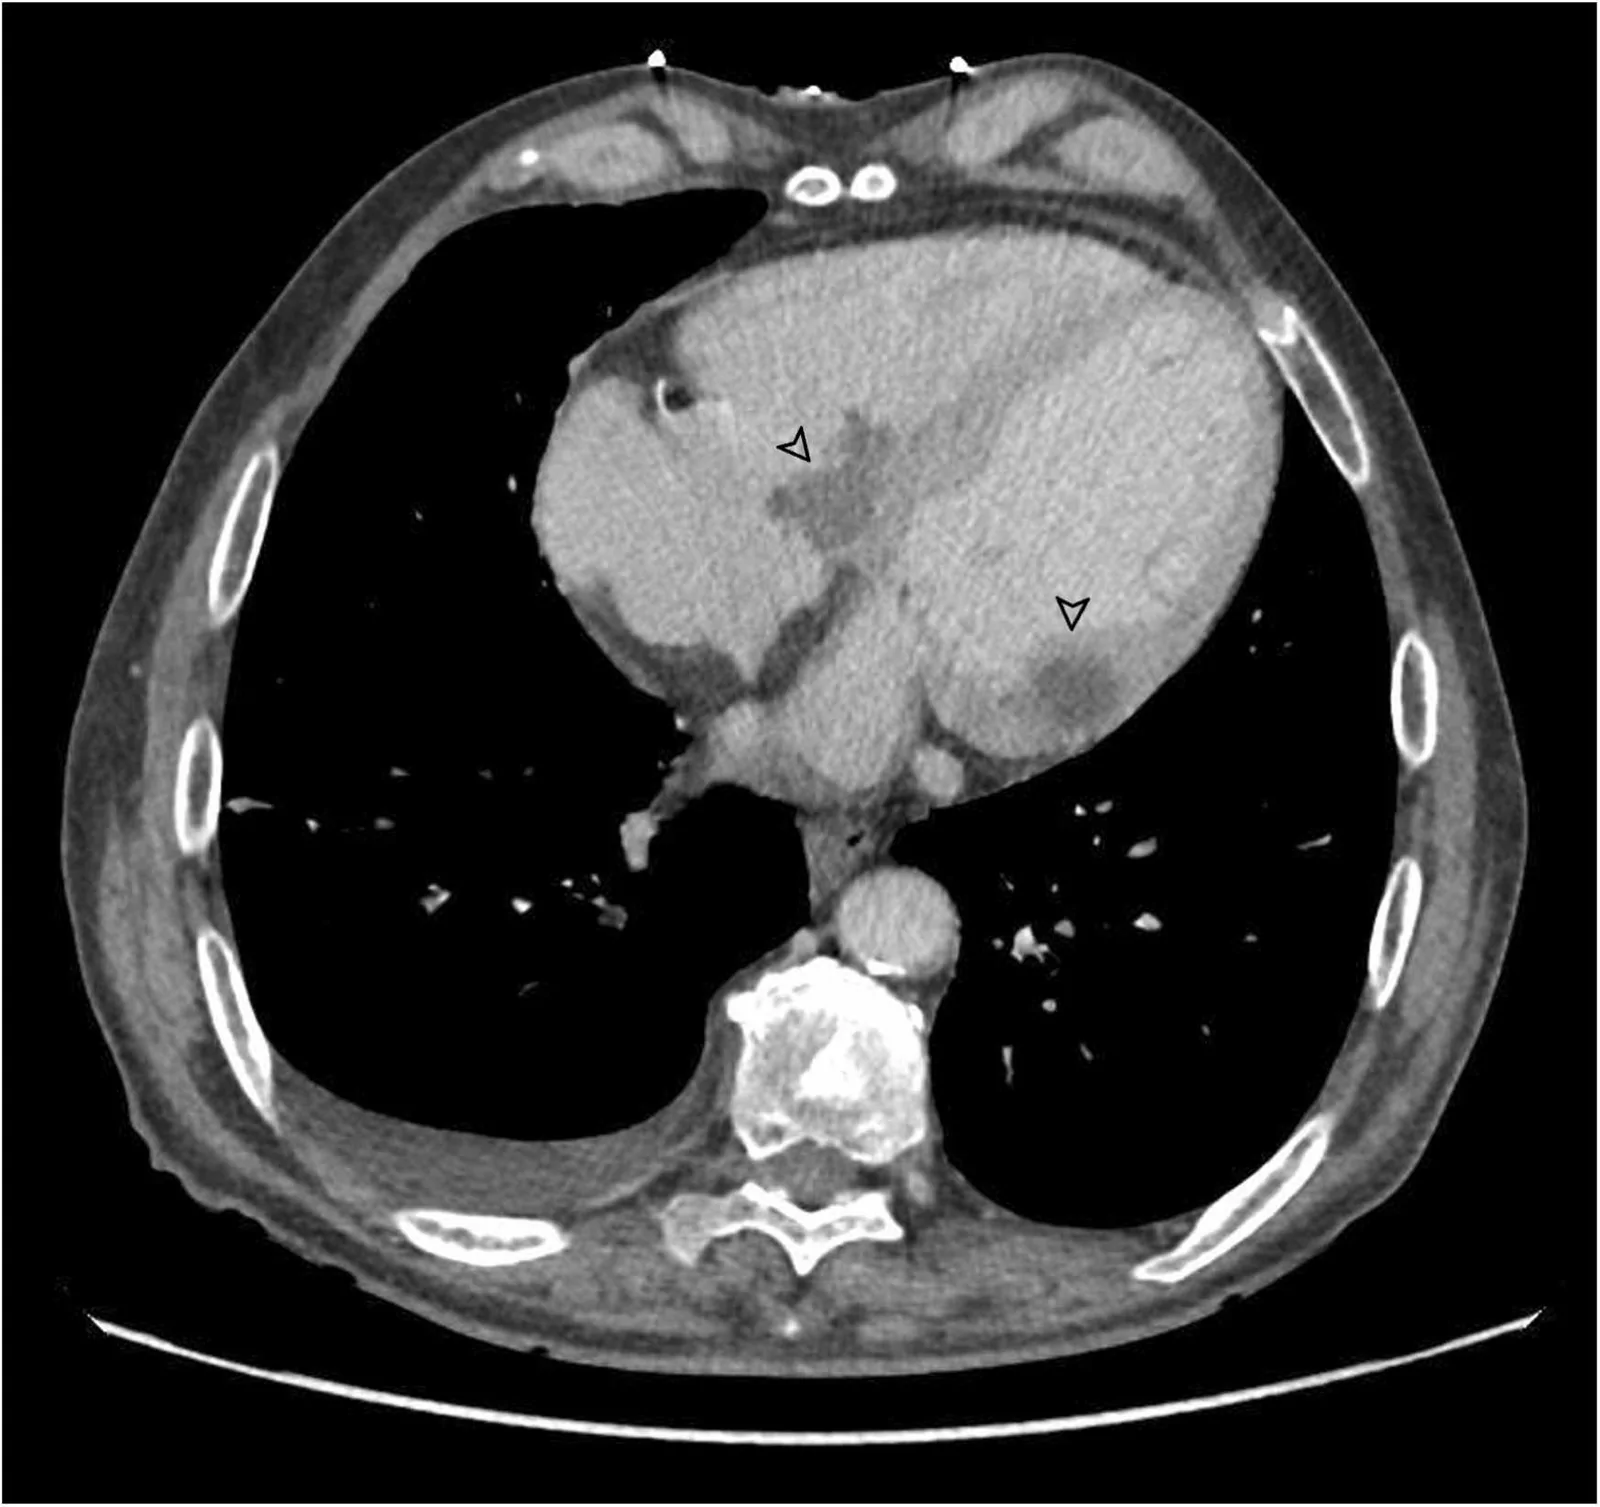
CT thorax with contrast showing multiple myometrial low-density lesions including within the interventricular septum and left ventricular wall (marked with black outlined arrows).
